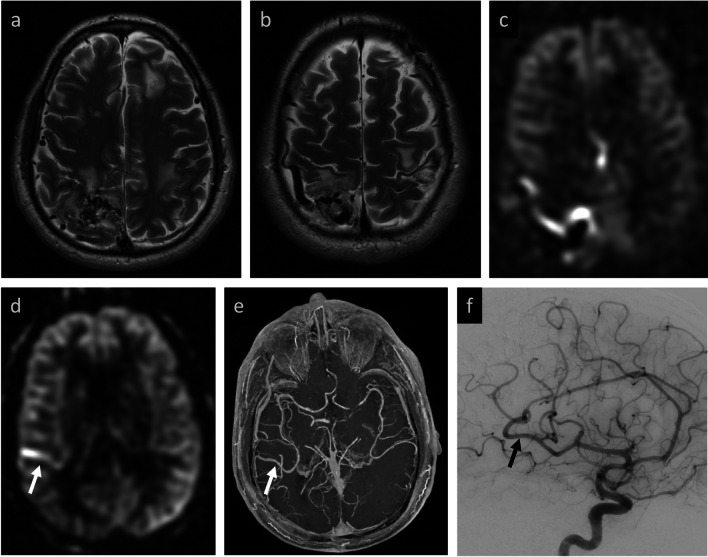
Example of inter-rater disagreement in evaluation of AVM treated with staged embolization and surgical resection. Baseline MRI: tangle of vessels in the right parietal lobe (a) and large draining vein (b), with corresponding ASL signal demonstrating AV shunting (c). Post-treatment MRI: After one additional embolization and subsequent surgical resection, curvilinear ASL signal inferior to the resection (white arrow, d) was thought to reflect residual shunting by one reader. On consensus review, both readers agreed that this likely reflects ASL signal in an ectatic distal MCA branch (white arrow, e) rather than residual shunting. Post-treatment DSA: lateral projection confirms a mildly ectatic MCA branch (black arrow, f), without residual AVM or early venous drainage
